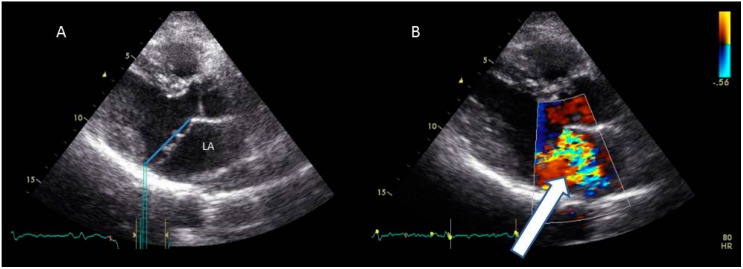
A parasternal long axis view of a prolapsing valve. Panel A shows the prolapsing valve relative to the annular line (blue line). Panel B shows color Doppler through the prolapsing valve demonstrating a severe jet of regurgitation (arrow). LA = left atrium.
